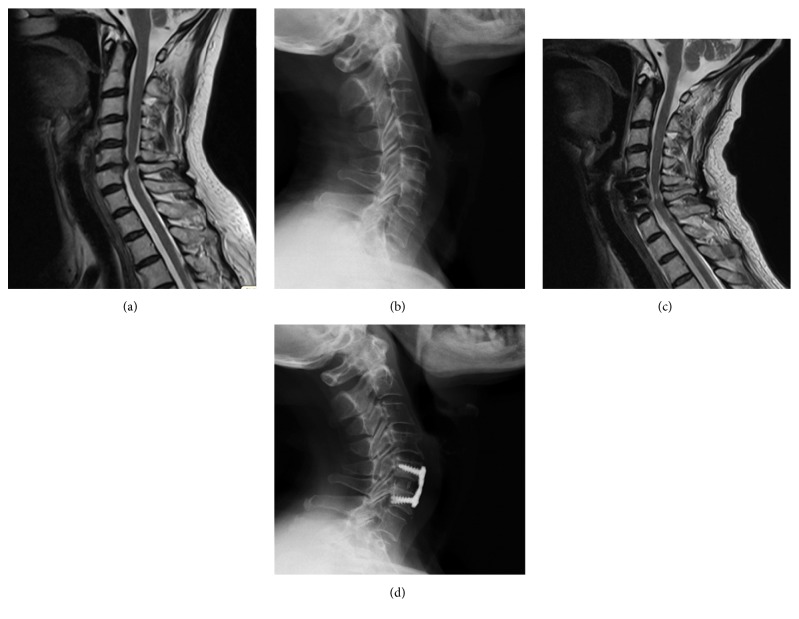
Female, 75-year-old, numbness of right limb, and difficulty in walking for 1 year. Preoperative JOA score was 12. The cervical MR scanning showed C5/6-disc herniation with posterior wrinkled and incrassated ligament Flava, leading to pincer spondylotic myelopathy. Anterior and posterior occupying rates were 36.4% and 36.4% (a). The lateral cervical X-ray showed intervertebral height loss in C5/6. Preoperative Cobb's angle was 9.7° (b). The posterior compression was relieved at the same time by anterior decompression and fusion (ACDF), and the spinal cord was decompressed sufficiently (c). The intervertebral plate and body height were increased after the operation. Postoperative Cobb's angle was 29.1° (d). Postoperative JOA score was 17 at 6th-month follow-up.
